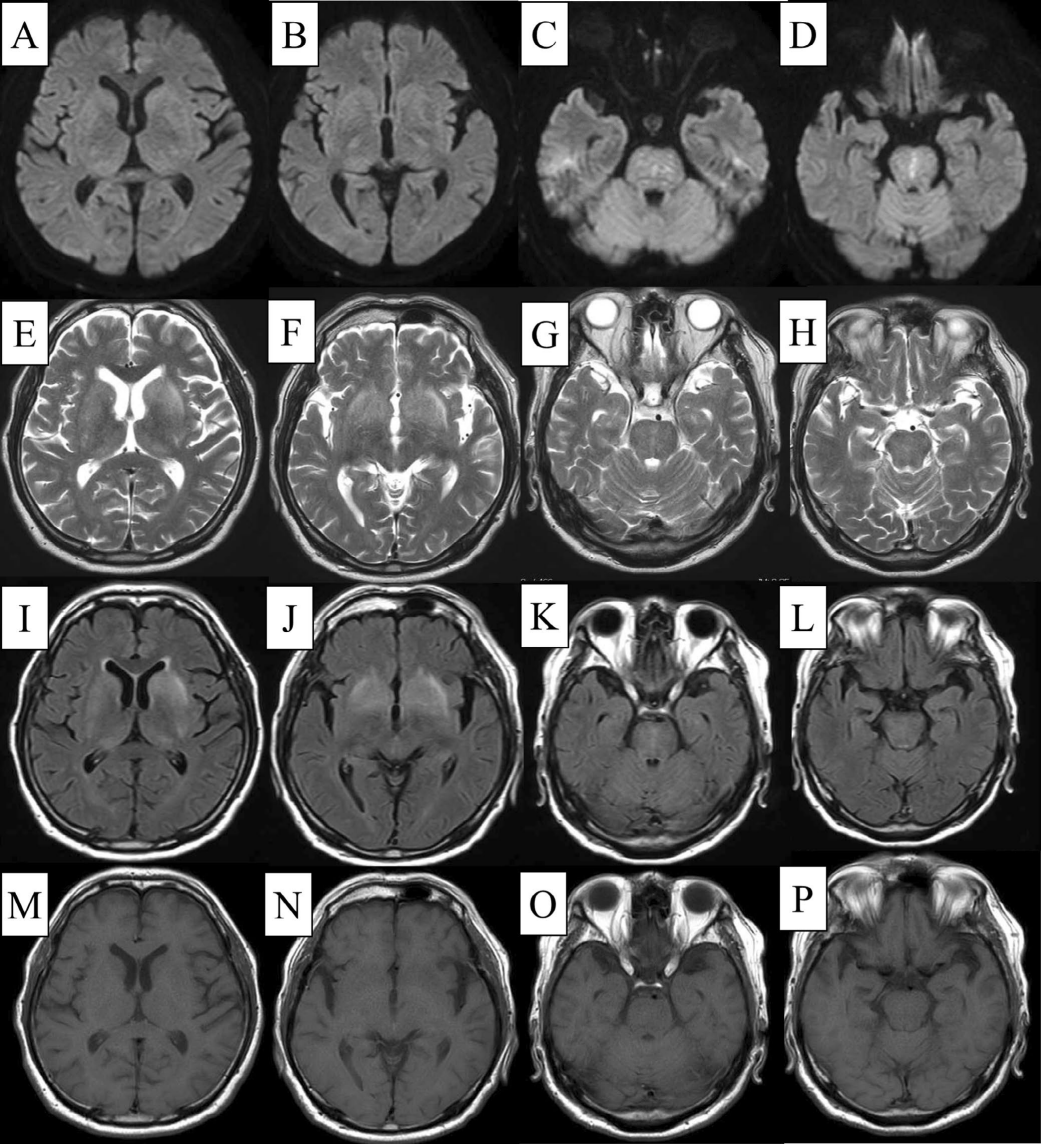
Head magnetic resonance image (horizontal section; A–D are DWI, E–H are T2WI, I–L are FLAIR, and M–P are T1WI). DWI, T2WI, and FLAIR images reveal symmetrical high signal intensities in the basal ganglia (A, E, I), external capsule, thalamus (B, F, J), and median part of the pons (C, G, K), with a predominance on the right side (D, H, L). No signal changes occurred on T1WI (M, N, O, P).
